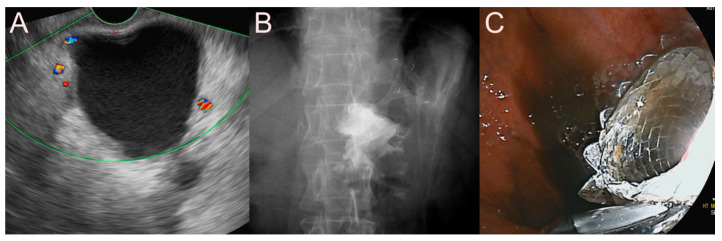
Ultrasound-guided pancreatic fluid collection drainage using the dedicated bi-flanged lumen-apposing metal stent (LAMS) (Niti-S SPAXUS; Taewoong Medical Co., Ltd., Ilsan, South Korea) for a walled-off necrosis (WON). (A) Endosonographic image showing a WON with heterogeneous necrotic debris. (B) Fluoroscopic image showing the LAMS placed between the stomach and the WON. (C) Endoscopic image showing the drainage of pus-like materials and large amounts of sludge through the LAMS.
